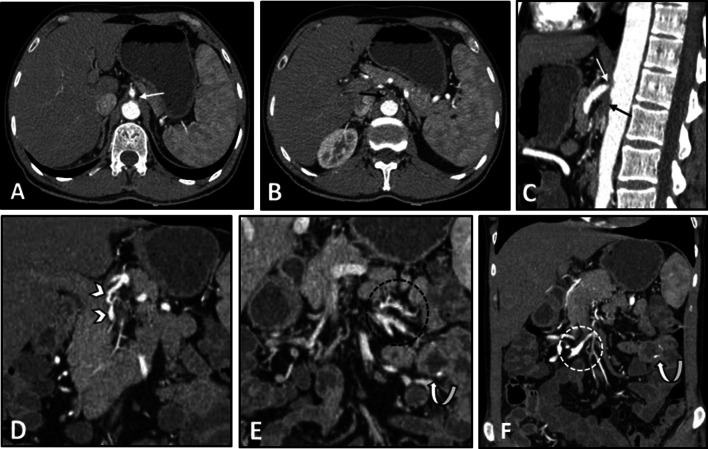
Vasculitis of splanchnic arteries in a 43-year-old male with a history of pathologically proven polyarteritis nodosa and segmental jejunal resection due to ischemia. He complains of postprandial abdominal pain, nausea, vomiting, hypertension, and elevated inflammatory markers. CTE was done. Axial and sagittal images (A–C) in the arterial phase demonstrate severe stenosis at the origin of the celiac trunk (thin white arrows) and complete occlusion and irregularity of proximal SMA (thin black arrows). Coronal CT images (D–F) reveal vessel irregularity and mild degree of aneurysmal dilations in a collateral branch between celiac trunk and SMA (white arrowheads), thickening and irregularity in jejunal branches of SMA (black dotted oval) adjacent to the previous site of jejunal resection and anastomosis (white curved arrows). The ileocolic branch of SMA also shows focal aneurysmal dilatation and mild thickening (white dotted oval)
